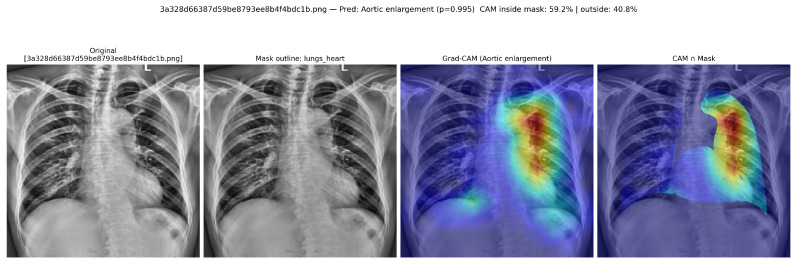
Anatomy-aware Grad-CAM for an aortic enlargement example. From (left) to (right): original chest radiograph, lungs and heart mask outline, Grad-CAM for the target class, and Grad-CAM restricted to the lungs and heart mask. Color overlays indicate activation intensity. The letter “L” denotes the left side of the chest radiograph. Inside-mask CAM energy is 59.2% (40.8% outside-mask).
